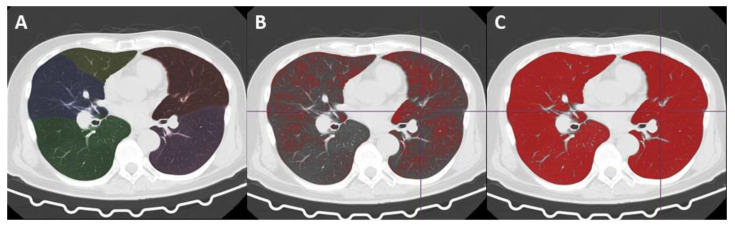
Automated volume analysis of the CT images. Chest computed tomography (CT) image of a 73-year-old man with suspected pulmonary hypertension. Automatically extracted and segmented lungs and lobes are color-coded (A). Red-painted areas indicate lung parenchyma with and under −950 HU (B) and −750 HU (C), which amounted to 21.7% and 91.6% of the whole lung (whole volume, 6456.6 mL; average density, −885.1 HU), respectively. Therefore, this patient’s %high attenuation area (from −749 HU to 0 HU) was calculated as 8.4% of the whole lung.
